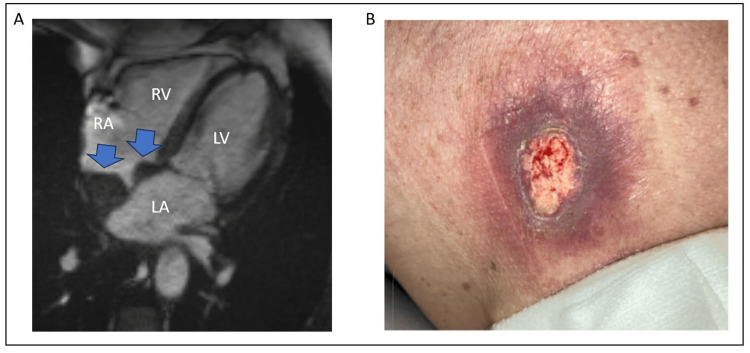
Cardiac MRI and skin lesion A – Cardiac MRI showing a large mass extending across the inter-atrial septum (blue arrows). B – Ulcerating skin lesion on the patient’s back. RA = right atrium; LA = left atrium; RV = right ventricle; LV = left ventricle.
